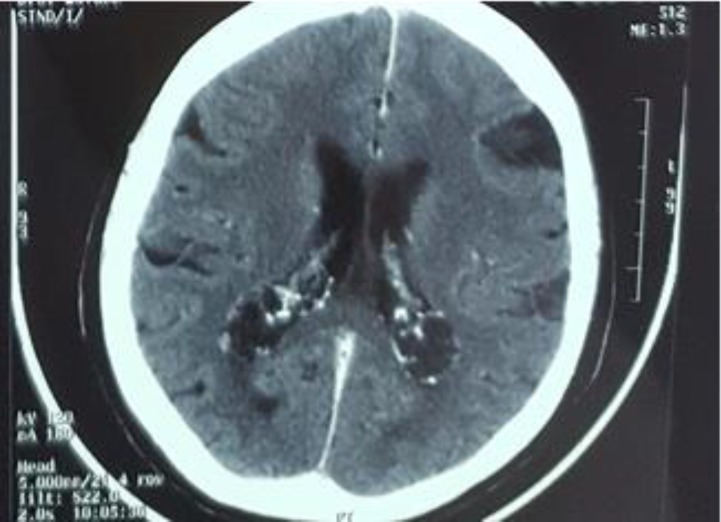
CT scan of the brain after irradiation
